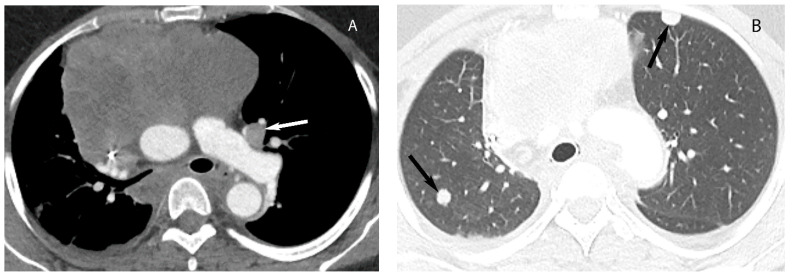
Thymic carcinoma. CT with soft tissue (A) and lung (B) window show large heterogeneous soft tissue mass in the prevascular mediastinum. Left prevascular lymphadenopathy (arrow in (A)) and well-marginated lung nodules (arrows in (B)) are compatible with metastatic disease.
